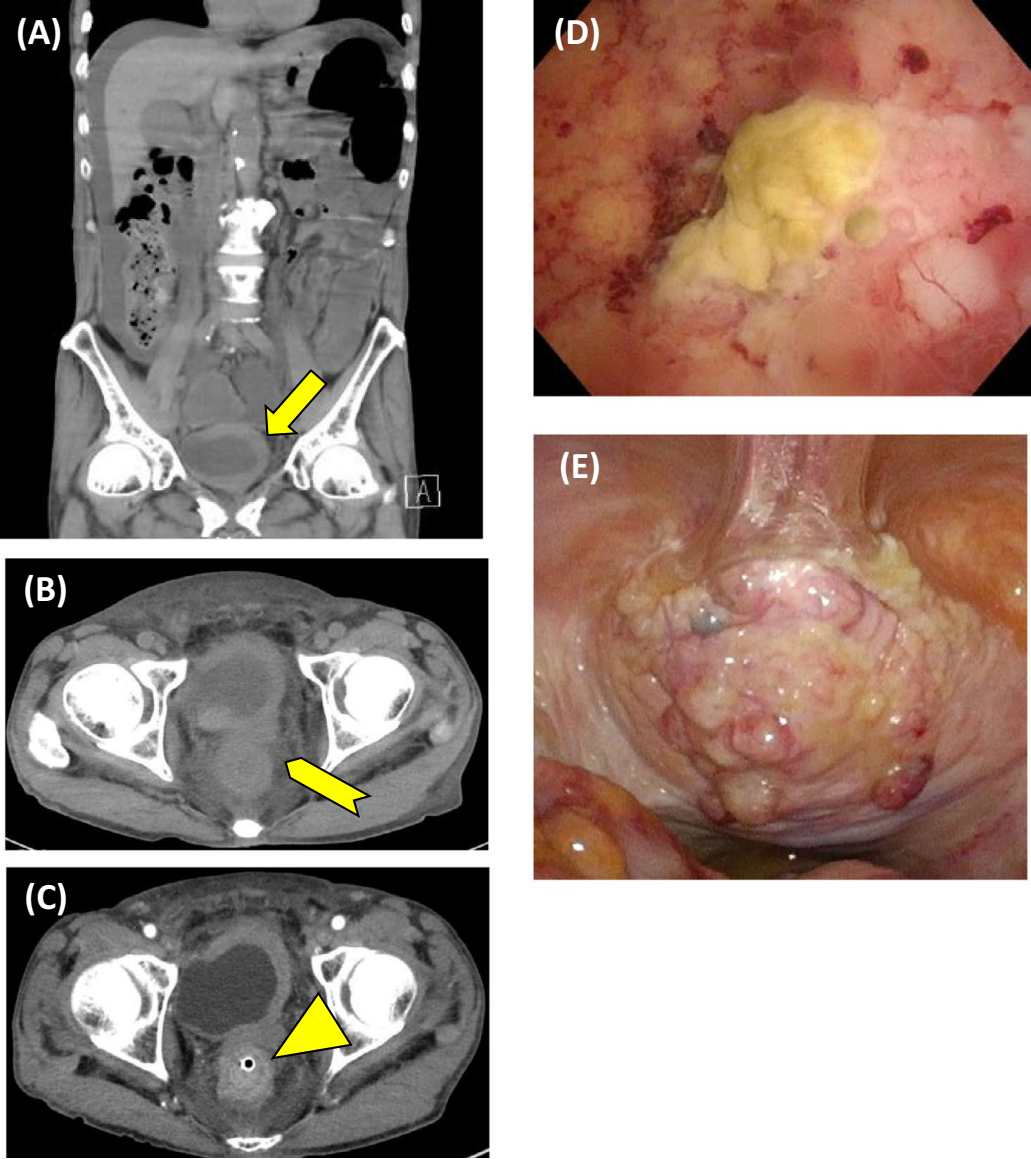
Abdominal CT revealed a bladder tumor extending from the dome to the left lateral wall, with suspected muscle invasion and left hydronephrosis (A, arrow), along with increased perirectal fat density, rectal wall thickening causing obstruction, and ascites (B, arrowhead). Contrast‐enhanced CT on hospital day 3 revealed the rectal wall alternating hypo‐ and hyperenhancement (C, triangle). Cystoscopy showed a partially necrotic mass predominantly involving the bladder dome (D). Tumor invasion extending to the peritoneum was apparent (E).
